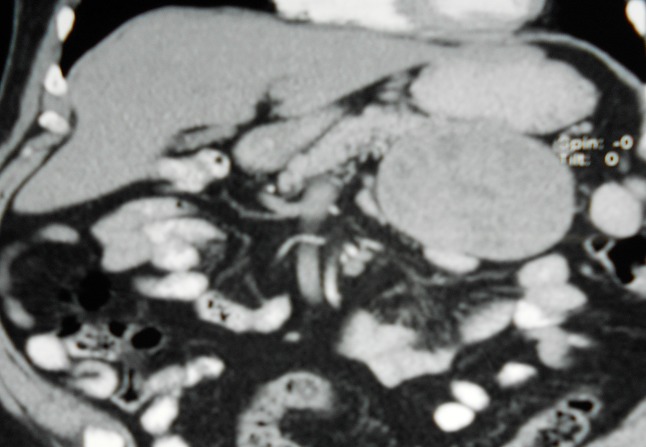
CT scan: well-marginated, encapsulated, heterogeneous mass, at the tail of the pancreas
